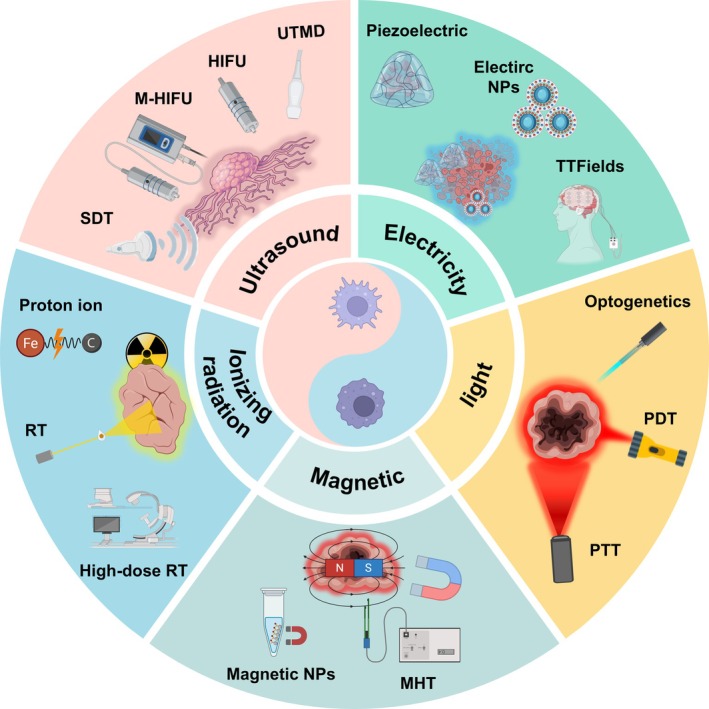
Non‐invasive physical stimulation modulates TAM polarization. Non‐invasive physical stimuli, including electrical, light, magnetic, ionizing radiation, and ultrasound stimulation, can regulate TAM polarization from a pro‐tumoral M2‐like phenotype toward an antitumoral M1‐like phenotype. These stimuli act through mechanosensitive receptors, ion channels, or metabolic reprogramming, triggering downstream signaling cascades that reshape the TME and enhance immunotherapy efficacy. TAM, tumor‐associated macrophage.
